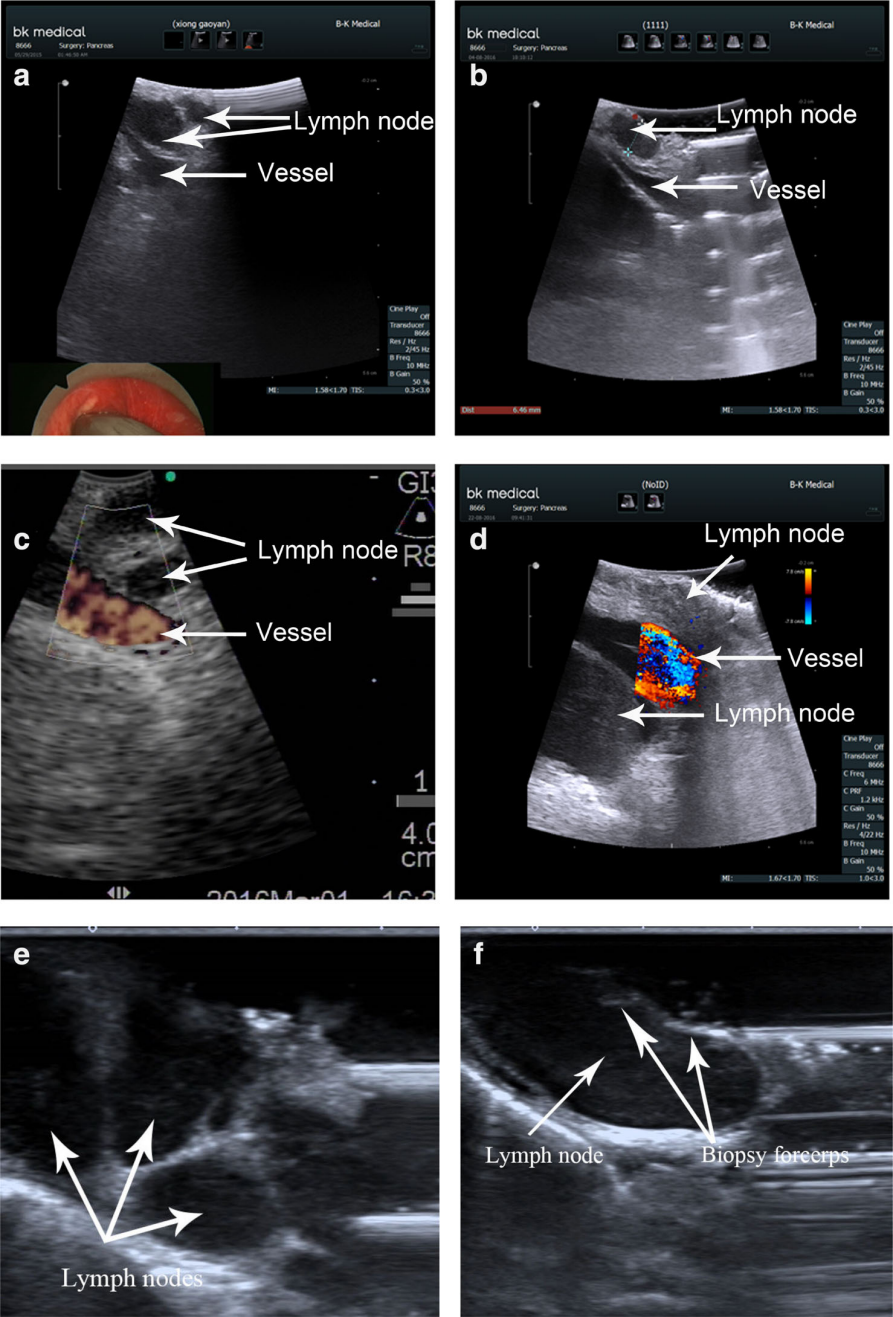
Ultrasonic measurement of mediastinal lymph nodes. (a, b) 2R lymph nodes behind a vessel. (c, d) Ultrasound assessment of the blood flow of lymph nodes, with clear distinction between lymph nodes and surrounding blood vessels. (e) Multiple lymph nodes visualized on ultrasound. (f) Biopsy forceps visualized on ultrasound.
